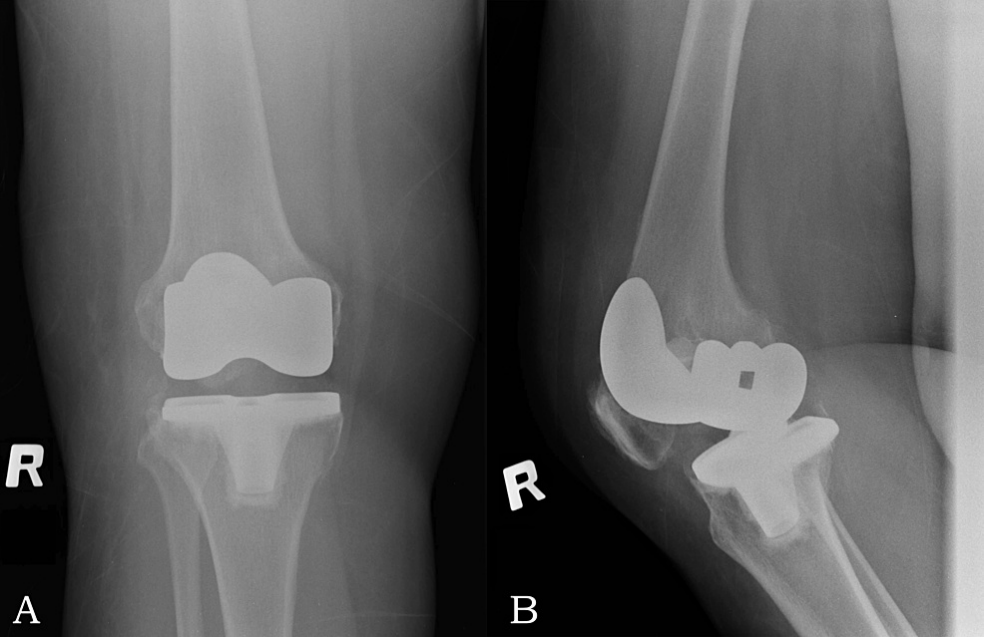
Plain radiographs of the right knee Anteroposterior view (A) and lateral view (B) showing no evidence of implant loosening.
